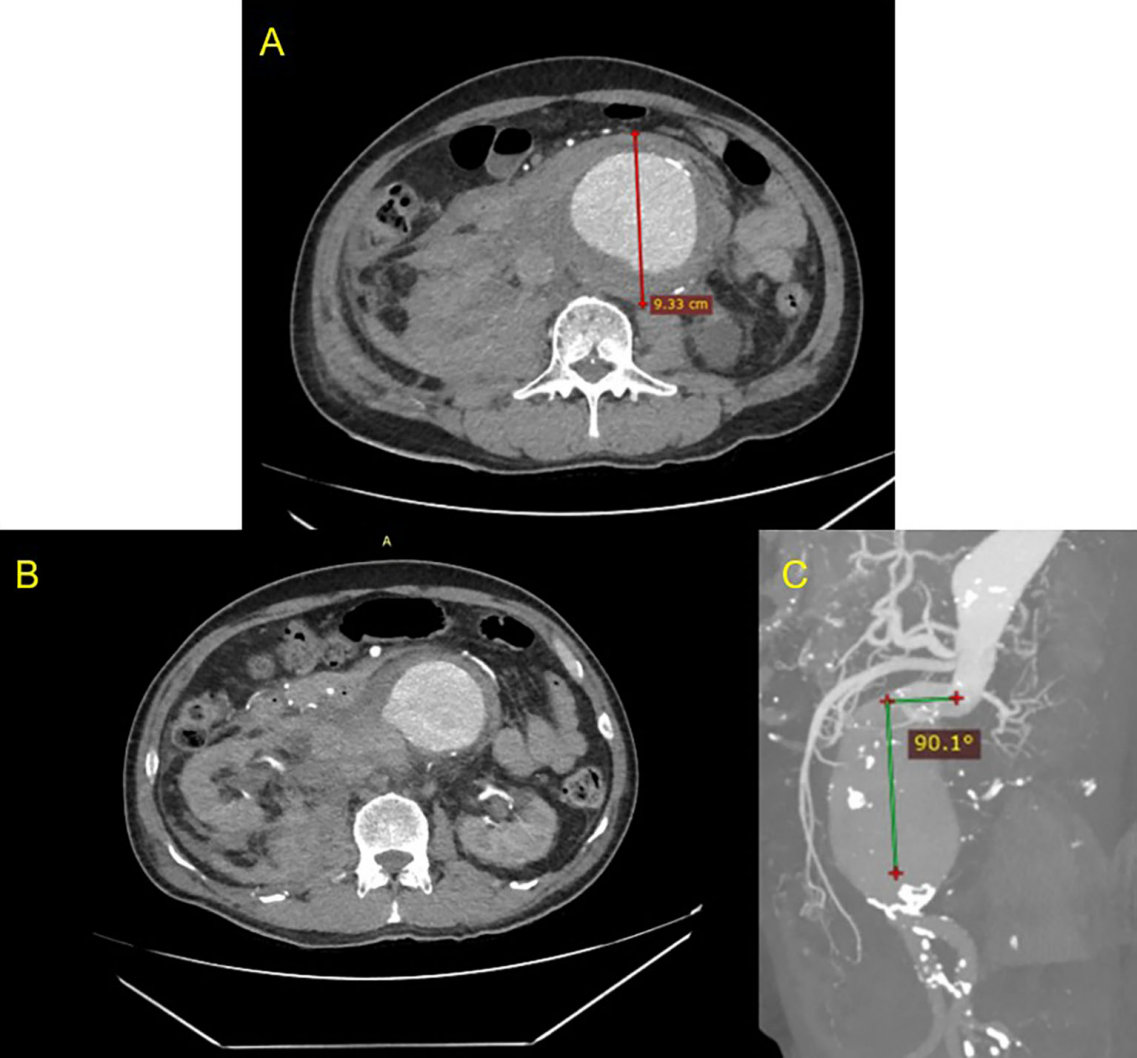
CT Scan of the patient before intervention. A. Pre-procedural axial slice CT Scan angiography showing giant abdominal aortic aneurysm; B. Axial CT Scan angiography showing ruptured aneurysm; C. Maximum Intensity Projection of CT Scan showing anatomy features of the conical neck and severe angulation (90.1°)
